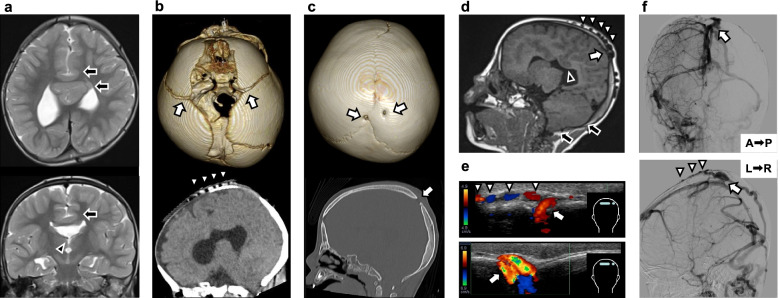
Imaging findings of syntelencephly, craniosynostosis, and sinus pericranii in the present case. a T2-weighted head MRI at age 2 years and 4 months. Abnormal midline connection between the cerebral hemispheres of the posterior frontal lobes and cingulate gyrus are shown (black arrow). The basal ganglia and thalamus are separated bilaterally (black arrow head). b Head computed tomography findings at age 4 months. The cranial sutures were not fused (white arrows). Tortuous and dilated subcutaneous veins were observed in the parietal region (white arrow heads). c Head computed tomography findings at age 2 years and 5 months. Two parietal skull bone defects (white arrows) and coronal craniosynostosis are visible. Digital impression on the skull was not evident. d T1-weighted head MRI at age 2 years and 4 months. Sinus pericranii (white arrow) via the parietal bone defect can be seen. The white arrowheads indicate distension of the subcutaneous veins in the parietal region. Callosal hypoplasia and ectopic gray matter around the lateral cerebral ventricles can also be observed. The black arrow indicates the tight posterior fossa and slight descent of the caudal part of the cerebellum toward the foramen magnum. Agenesis of the body of the corpus callosum and continuity of the cingulate gyrus across the midline are also shown. e Color cranial Doppler ultrasound findings at age 2 years and 5 months. Blood flow in two abnormal blood vessels (sinus pericranii) through the parietal bone defects to the extracranial veins can be seen (white arrow). The subcutaneous veins show distension (white arrow heads). f Cerebral angiography confirmed that the abnormal blood vessels formed a communication between the superior sagittal sinus and parietal diploic and subcutaneous veins (white arrows). No obvious thrombi, dural sinus abnormalities, or other vascular malformations were observed. The subcutaneous veins show distension (white arrow heads). L → R, left–right view (upper); A → P, anterior–posterior view (lower)
